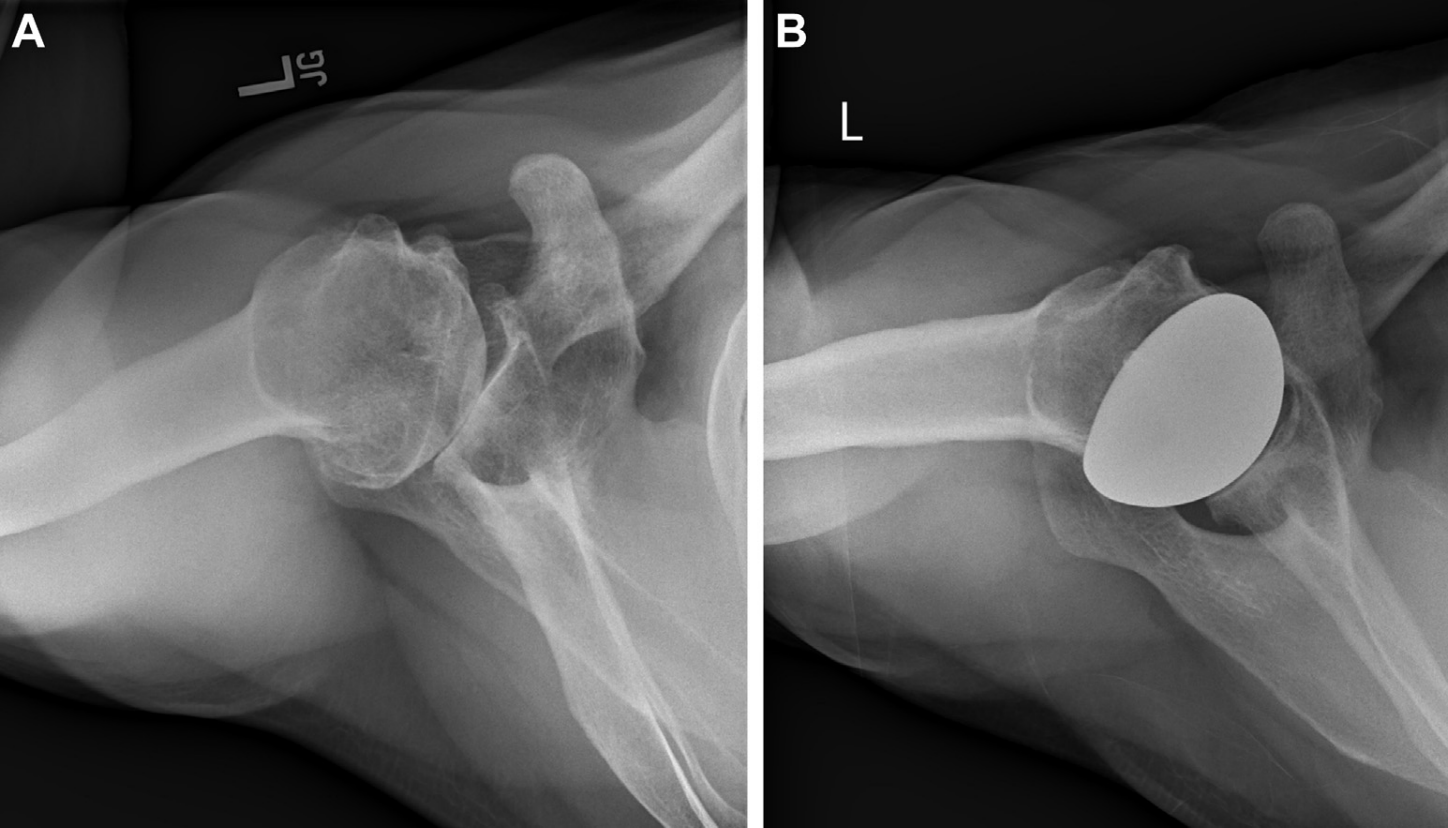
(A) Preoperative radiographic imaging. (B) Postoperative radiographic imaging.
